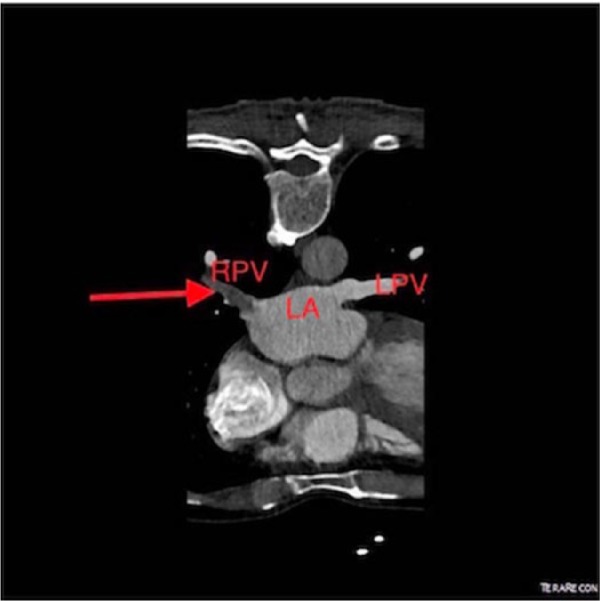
Computed tomography chest with pulmonary embolism protocol. Red arrow shows pulmonary vein thrombosis. RPV, right pulmonary vein; LPV, left pulmonary vein; LA, left atrium.
